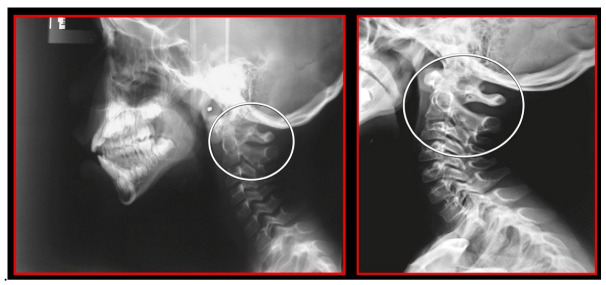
Rotation of the *atlas* on a lateral cephalometric radiograph. Left: Pre-treatment. Double ring of the *atlas* showing its rotation. Decreased functional spaces. Right: After orthopedic manual therapy. Only one posterior arch of the *atlas* showing the normalization of its rotation. Normalized functional spaces.
